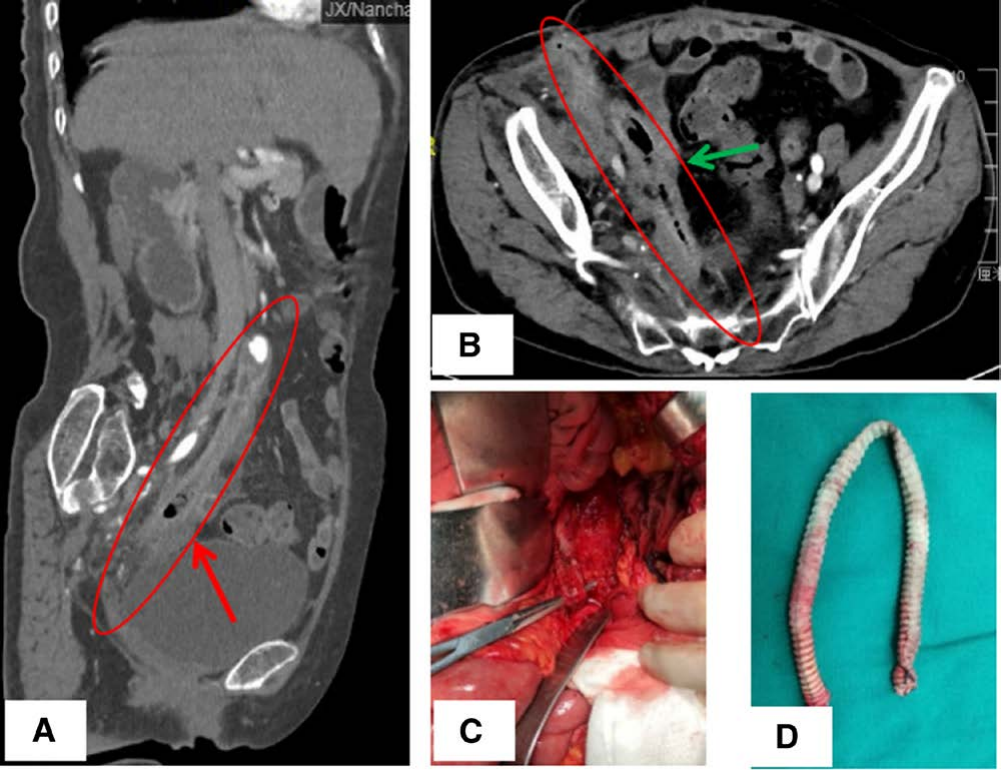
The CT examination and the integrity of infected artificial graft. (A, B) Before the second surgery (removal of vascular grafts), computed tomographic angiography (CTA) showed ectopic gas around the artificial blood vessels, and the right lower anterior abdominal sinus was formed. (C) Infected vascular grafts were found in the second operation. (D) Excised infected vascular graft at the second surgery. CTA = computed tomographic angiography.
